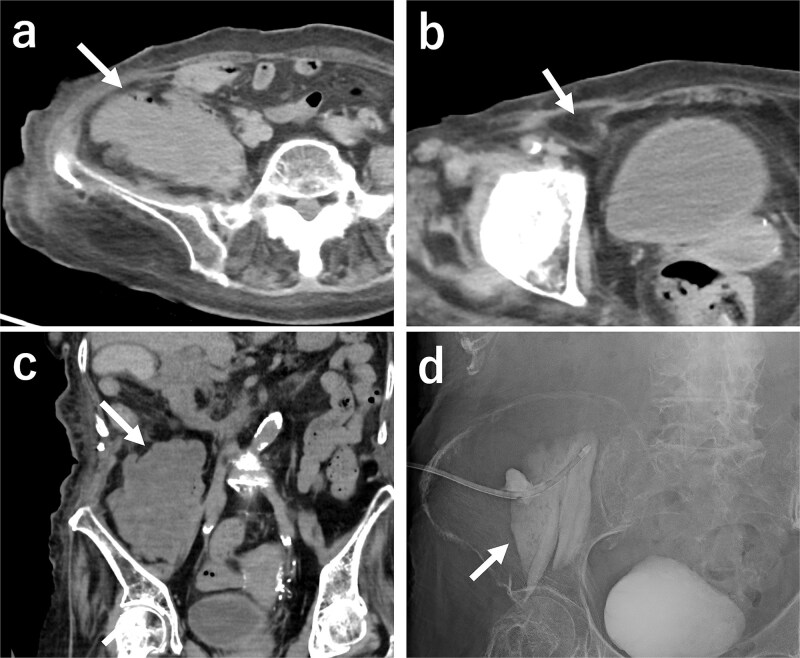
Imaging after readmission. (a–c) Abdominal CT. Fluid collection is present around the cecum but not in the groin area. (d) Contrast imaging of the abscess. No communication is evident between the abscess and the intestinal tract.
